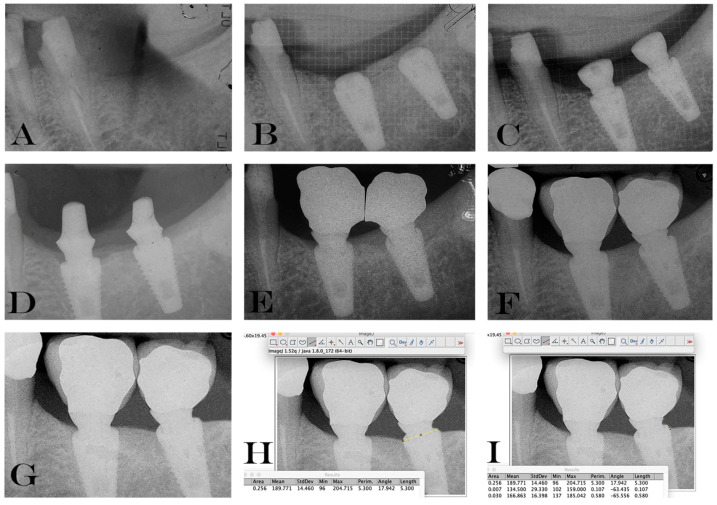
Periapical radiographs showing all the phases of the study (A–G): (A) pre-operative periapical radiography; (B) immediately after implant placement; (C) at the second surgical stage; (D) cementation of final abutments; (E) crown structure test; (F) delivery of the permanents metal-ceramic crowns; (G) radiograph taken 4 years after implant insertion. (H–I) demonstration of the MBL measurements with computer-assisted calibration to guarantee a correct measurement also in cases where the radiograph was slightly angulated.
